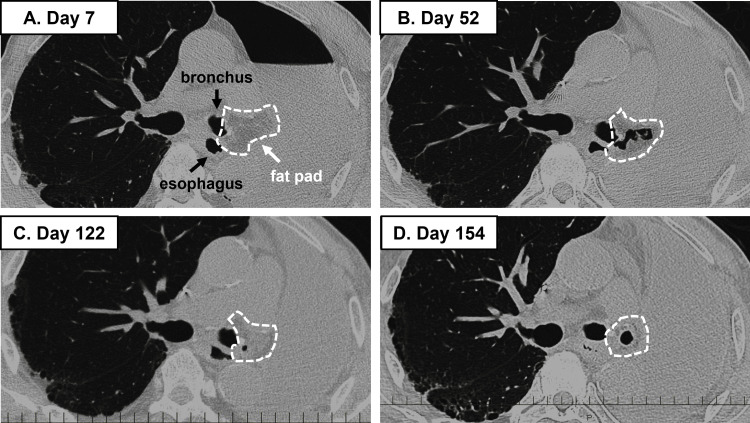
Air in the free pericardial fat covering the bronchial stump. (A) Day seven. (B) Day 52. (C) Day 122. (D) Day 154. Computed tomography performed 154 days postoperatively, at the onset of empyema, showed no evidence of aspiration pneumonia in the contralateral lung. The bronchial stump is outlined by uneven white dotted circles.
